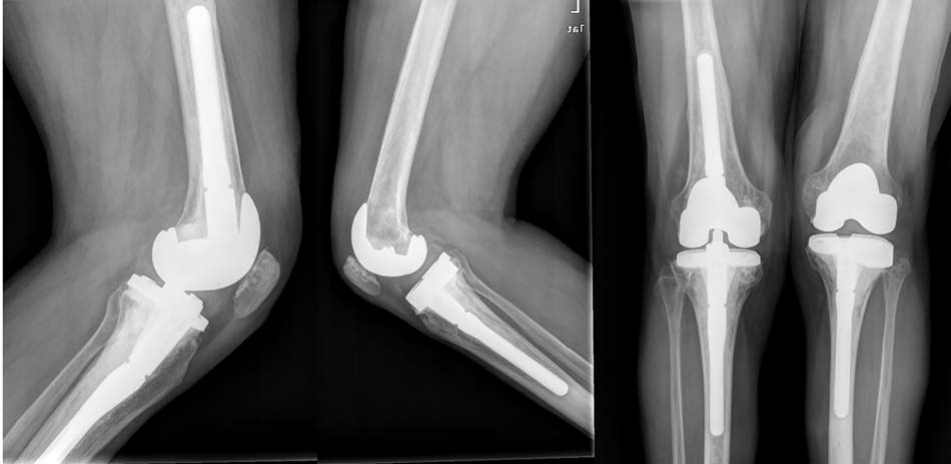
Both knees standing radiographs at 4-year follow-up with no signs of radiological loosening.
